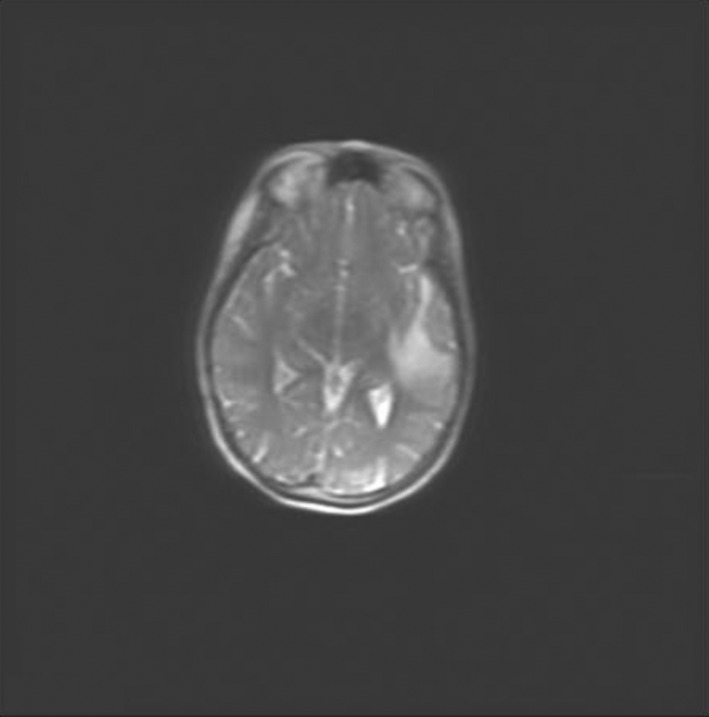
Magnetic resonance imaging at central nervous system lymphoma recurrence
